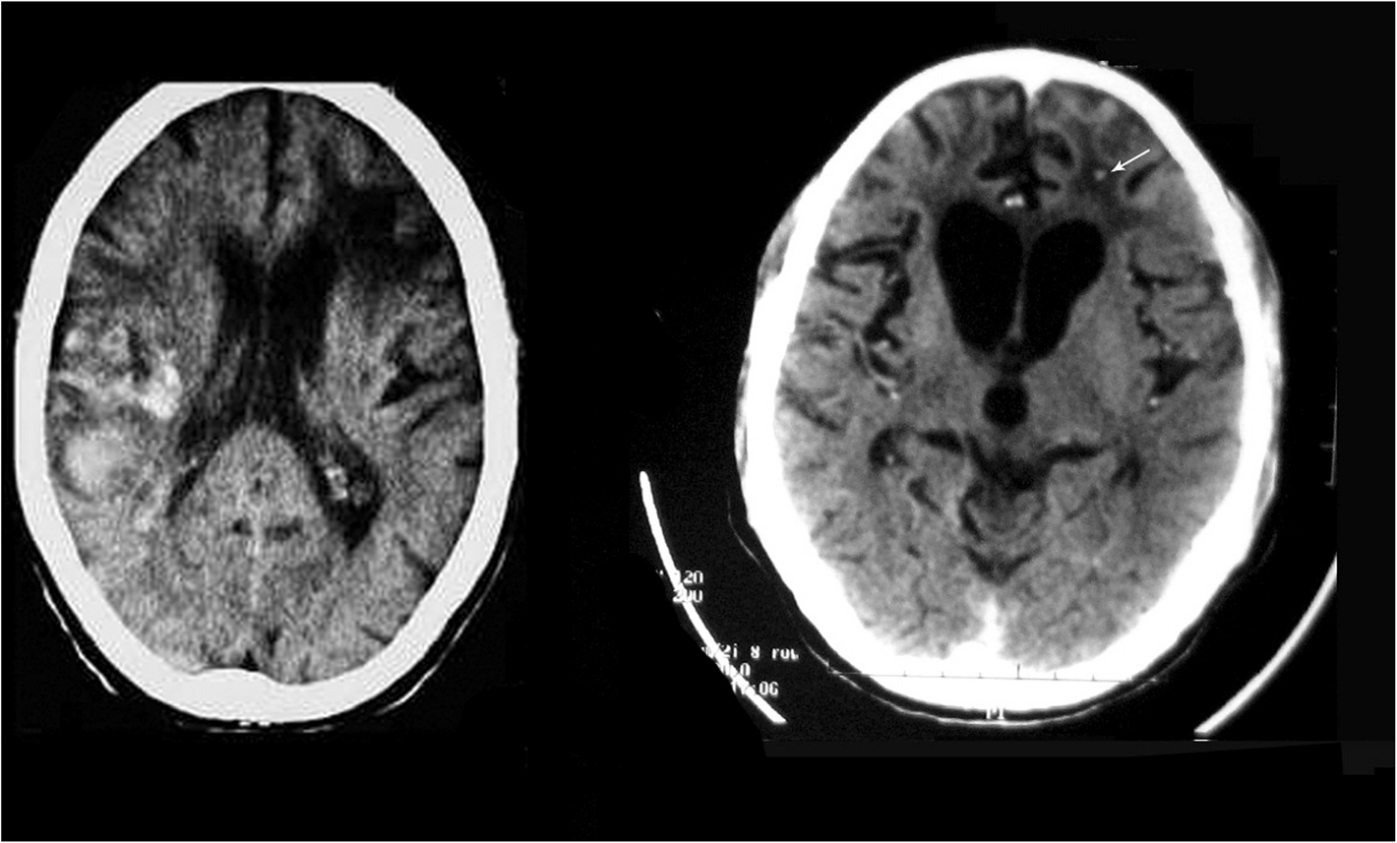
Repeat head computed tomography on the 15^th ^day of admission revealing resolving intraparenchymal hemorrhages (arrow).
